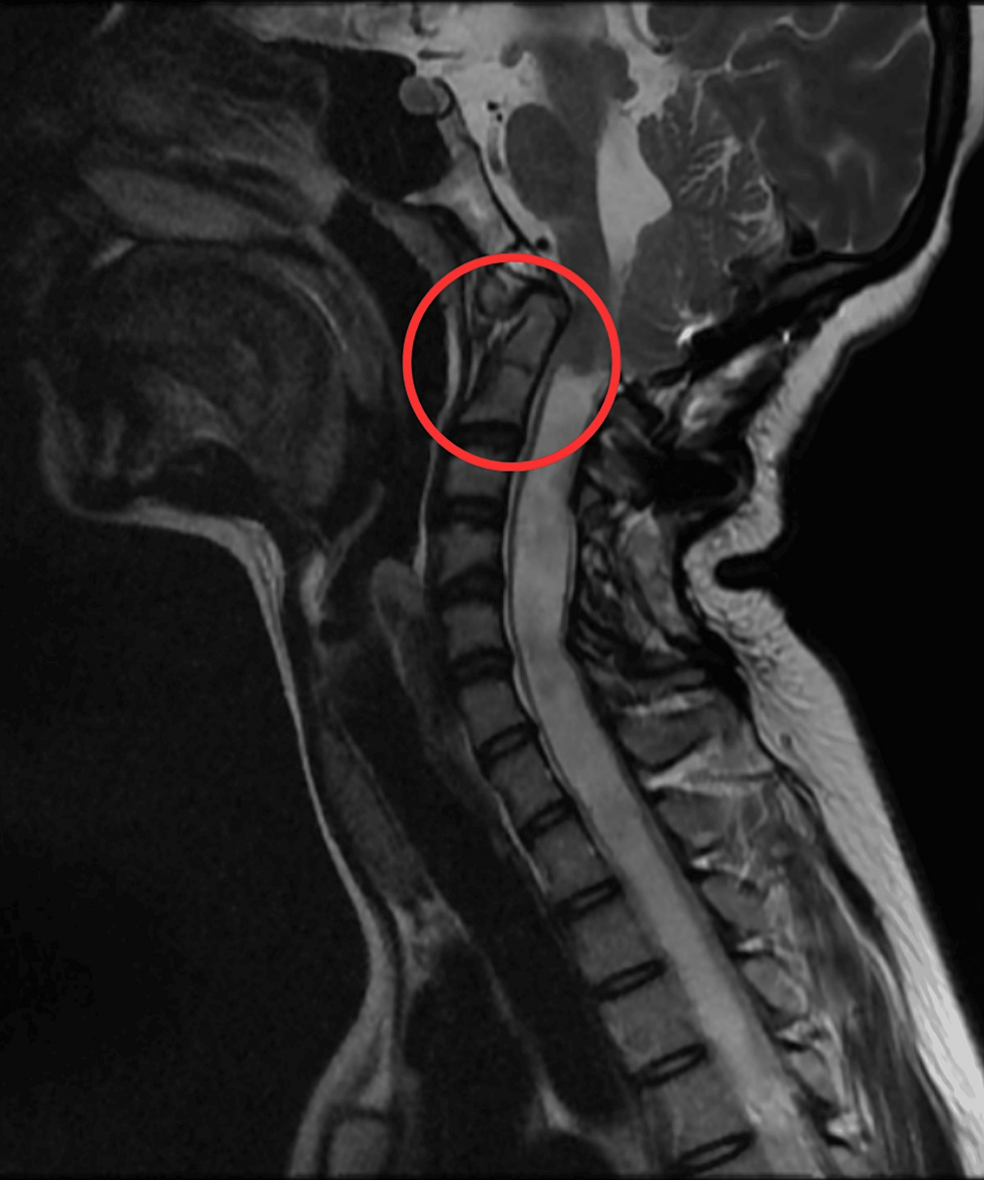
Cervical spine MRI The circle represents C2-C3 fusion and tonsillar herniation.
